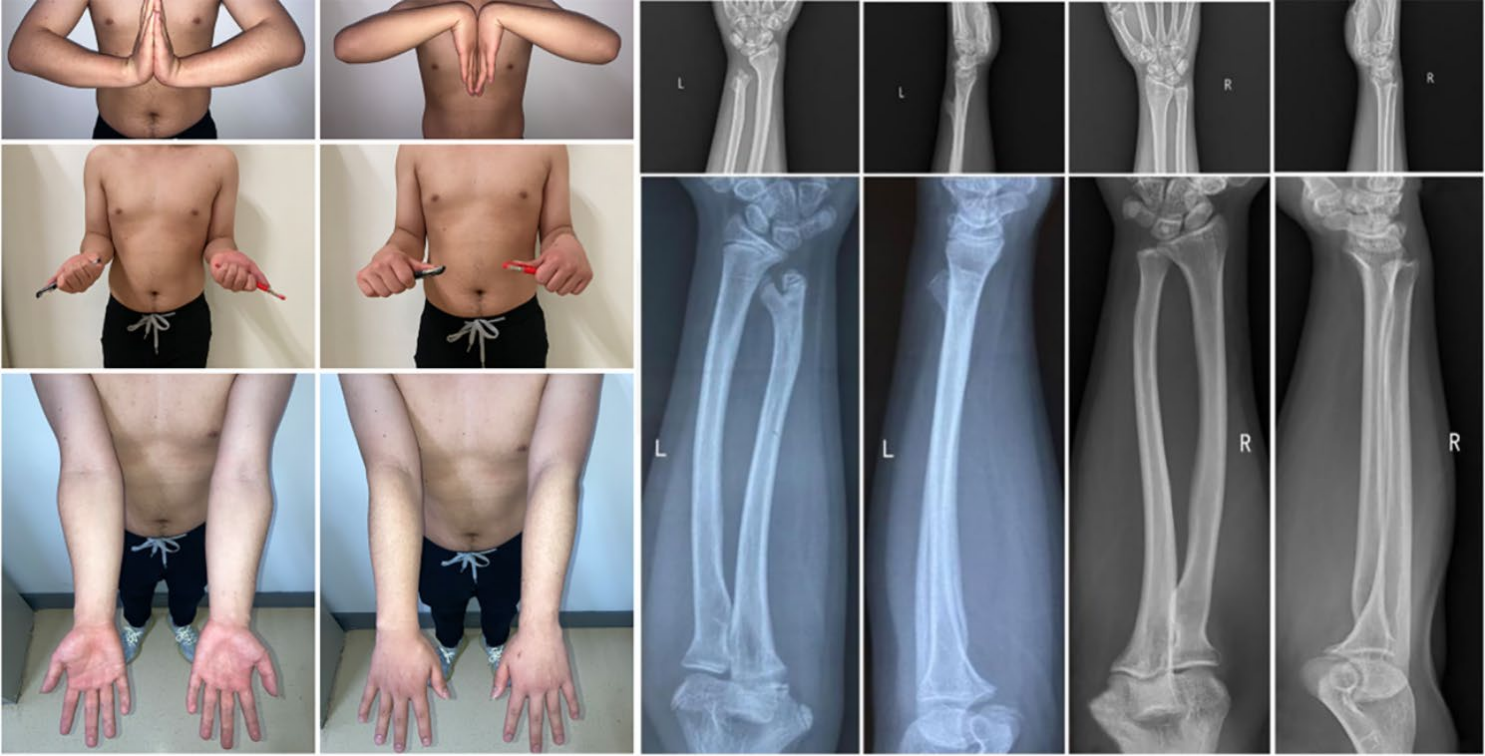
Movement and X-rays at 7 years of follow-up
